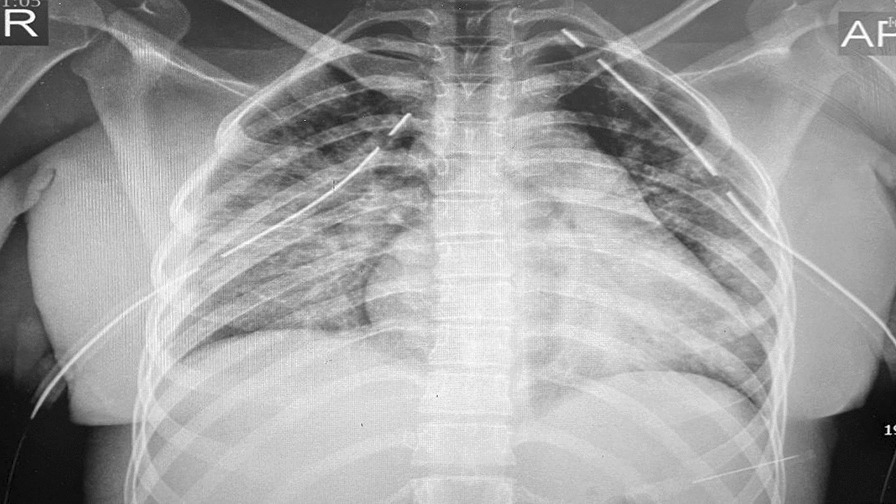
Anterior–posterior view chest x-ray following bilateral chest tube placement
